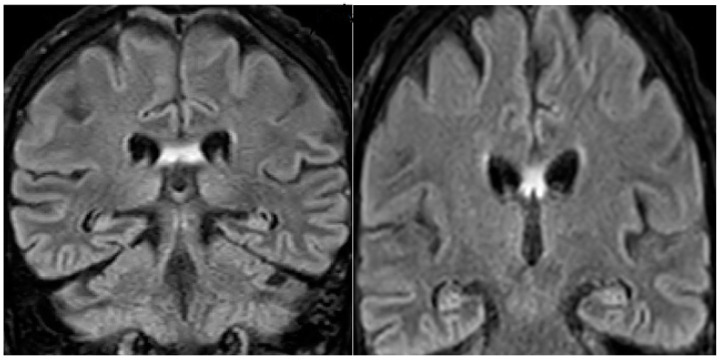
Brain MRI of the patient showing bilateral fornix hyperintensity in the FLAIR (fluid-attenuated inversion recovery) sequences.
